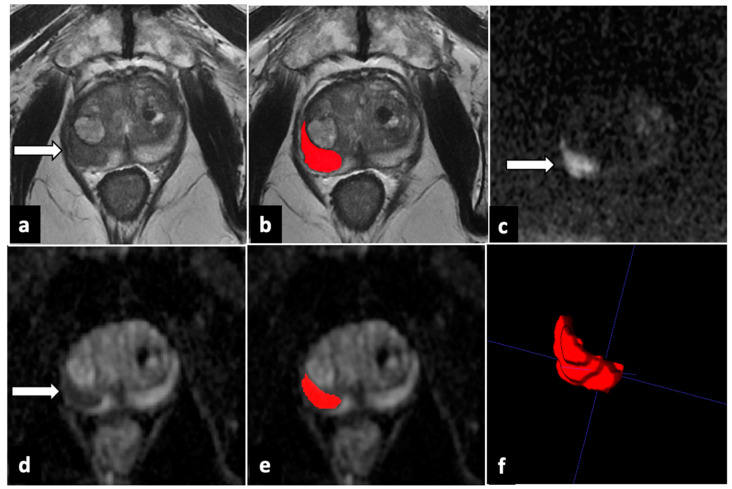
ROI delineation and generated 3D VOI in a peripheral zone lesion (*white arrows*). In the axial T2-weighted image (a), a markedly hypointense lesion exceeding 15 mm in length is observed in the anterior/posterior-lateral right peripheral zone of prostate midportion. The lesion showed high signal intensity in DWI (c) and low signal intensity in the ADC map (d). The lesion was scored as PI-RADS 5 and manually contoured on T2-weighted and diffusion-weighted images (b,e). A 3D VOI of the whole lesion (f) was also generated. ADC: apparent diffusion coefficient; DWI: diffusion-weighted imaging; PI-RADS: Prostate Imaging–Reporting and Data System; ROI: region of interest; 3D VOI: three-dimensional volume of interest. Red part shows the contouring and final volume obtained from the lesion.
